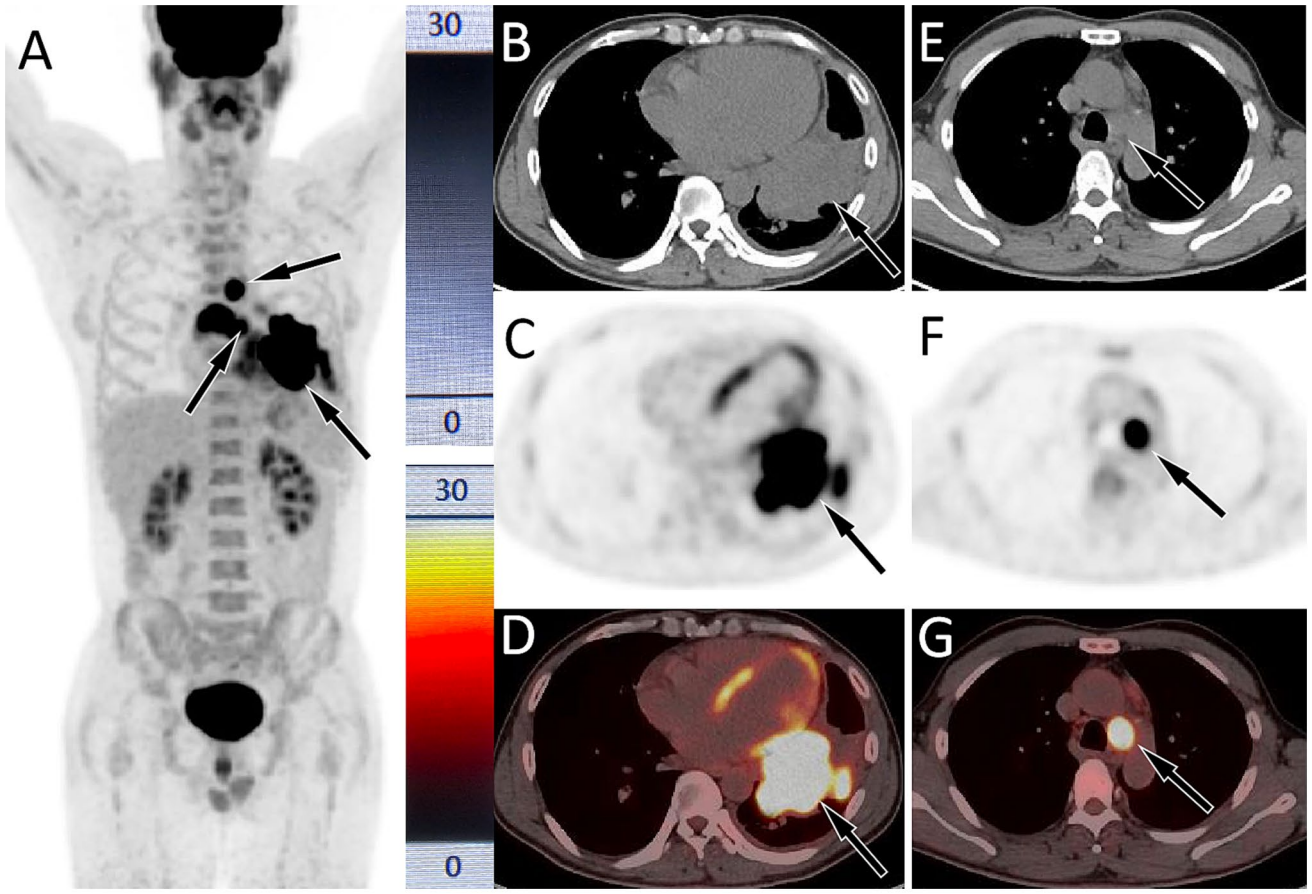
Fluorine-18 fluorodeoxyglucose (^18^F-FDG, with 314.5 MBq of injected activity) positron emission tomography (PET)/CT imaging of the patient on August 24, 2020; The maximum intensity projection (MIP, A) showed multiple significantly increased ^18^F-FDG uptake in the chest (arrows). Axial CT (B) showed a soft tissue density mass in the left portal area of the lung (arrow). The corresponding lesion had significantly increased ^18^F-FDG uptake on axial PET (C, arrow) and PET/CT fusion (D, arrow), with a maximum standardized uptake value (SUVmax) of 29.5. (E) Axial CT also showed an enlarged lymph node in the mediastinum, with a short-axis of about 2.0 cm; which was significantly increased ^18^FDG uptake on PET (F) and PET/CT (G), with a SUVmax of 25.3.
